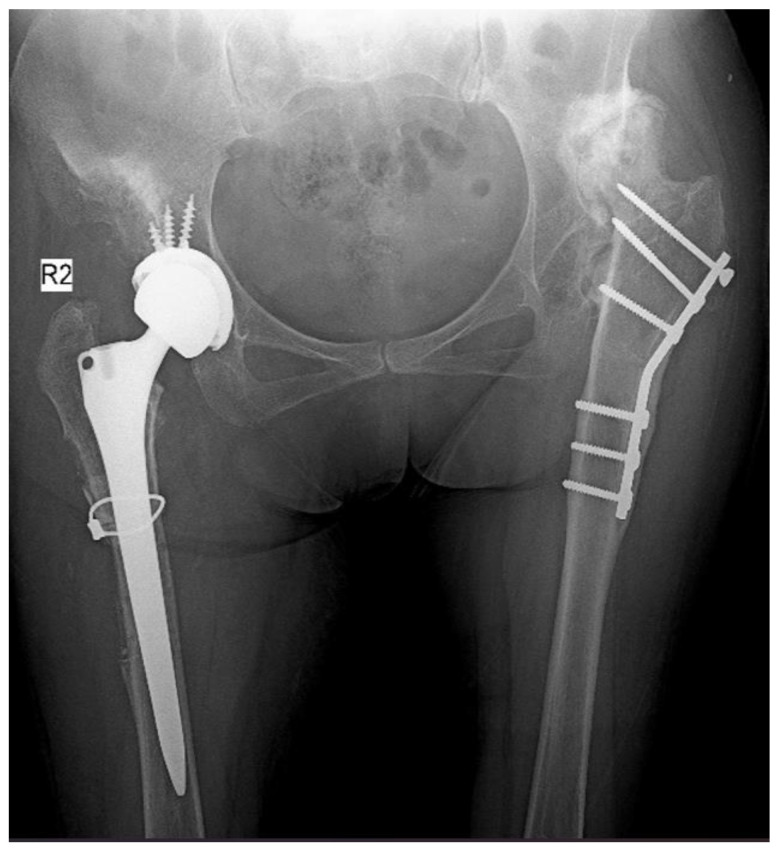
Postoperative X-ray image of the patient whose preoperative planning was performed using a robotically assisted surgical technique.
